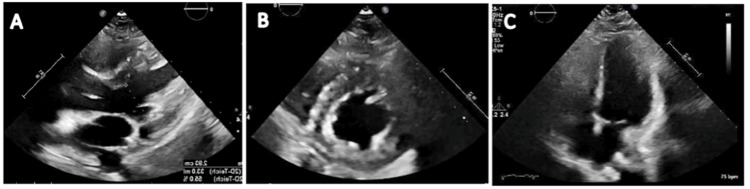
Transthoracic echocardiogram images showing parasternal long axis (A), parasternal short axis (B), and apical four chamber views (C). There is normal ejection fraction, normal left ventricular wall thickness, normal wall motion, systolic and diastolic functions.
